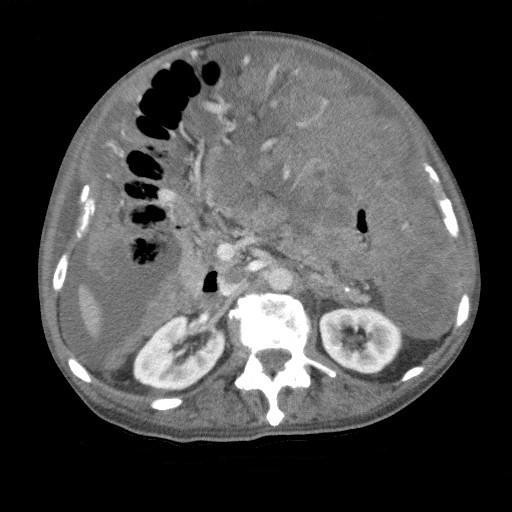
Axial 5 mm CT slice, at the level of the renal hilum, demonstrating dense omental caking, high density fluid with increased omental vascularity.
